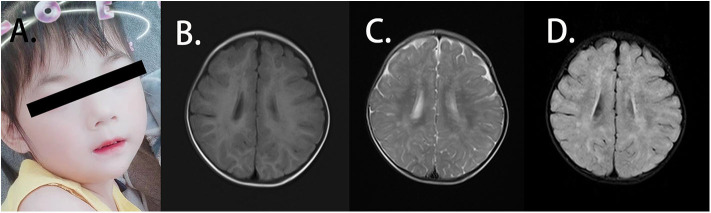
Clinical features of the patient. (A) The patient presented with facial dysmorphic features with hypertelorism, long philtrum, and thin upper lip vermilion. (B) Axial T1W1 magnetic resonance imaging (MRI) of the patient displayed blotch and piece-like lesions with the prolonged signal. (C) Axial T2W1 MRI imaging of the patient showed the abnormal shades distributed in bilateral frontal, temporal, parietal, and occipital lobes and basal ganglia. (D) From axial FLAIR imaging, the abnormal lesion was identified mainly in the white matter, and strip-like signals can also be observed in the bilateral basal ganglia.
